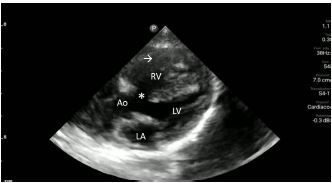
Parasternal long-axis view of the heart highlighting the findings of a ventricular septal defect (*) with an overriding aorta (Ao) and right ventricular hypertrophy (arrow). LA indicates left atrium; LV, left ventricle; RV, right ventricle.
